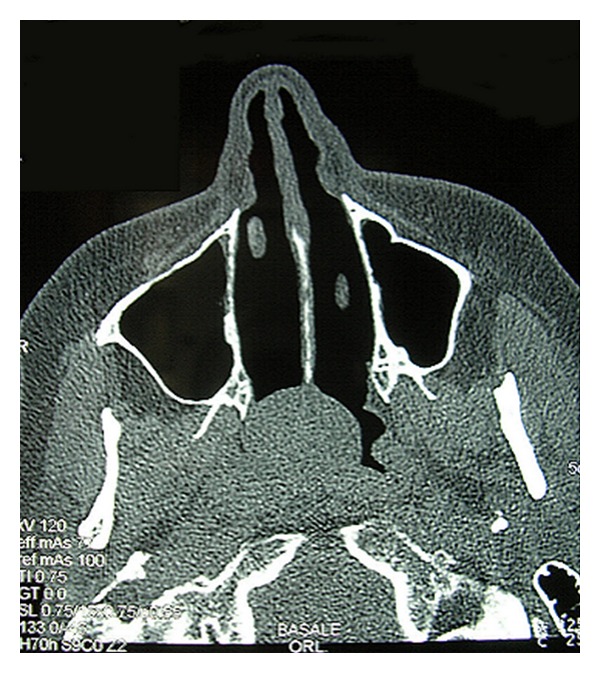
Axial CT scan: the polypoid mass occupying the rhinopharynx with no signs of bony framework erosion or infiltration.
